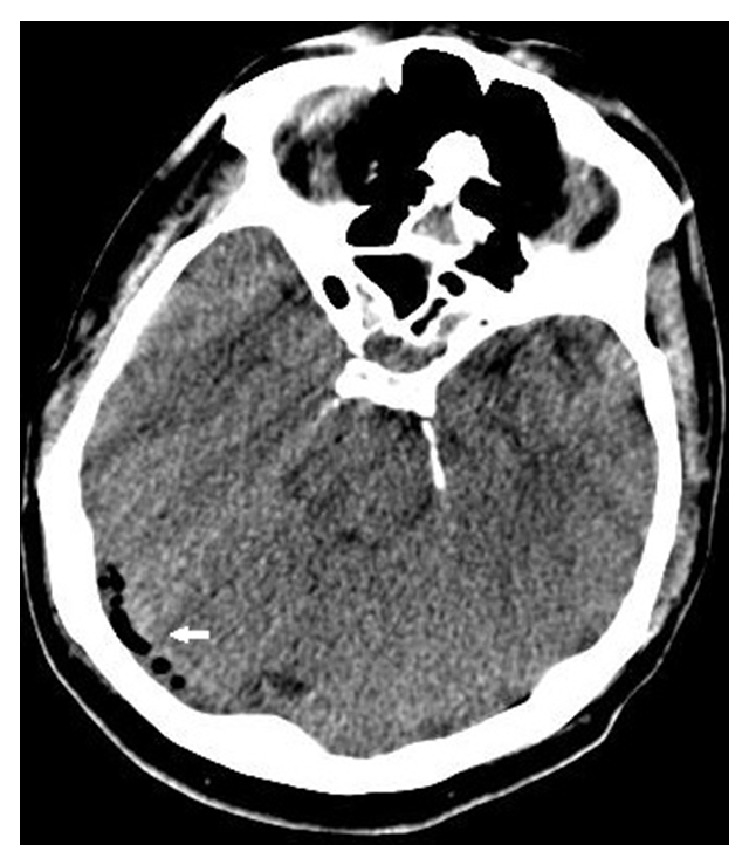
Air bubbles as seen in the brain CT of the right sigmoid sinus.
